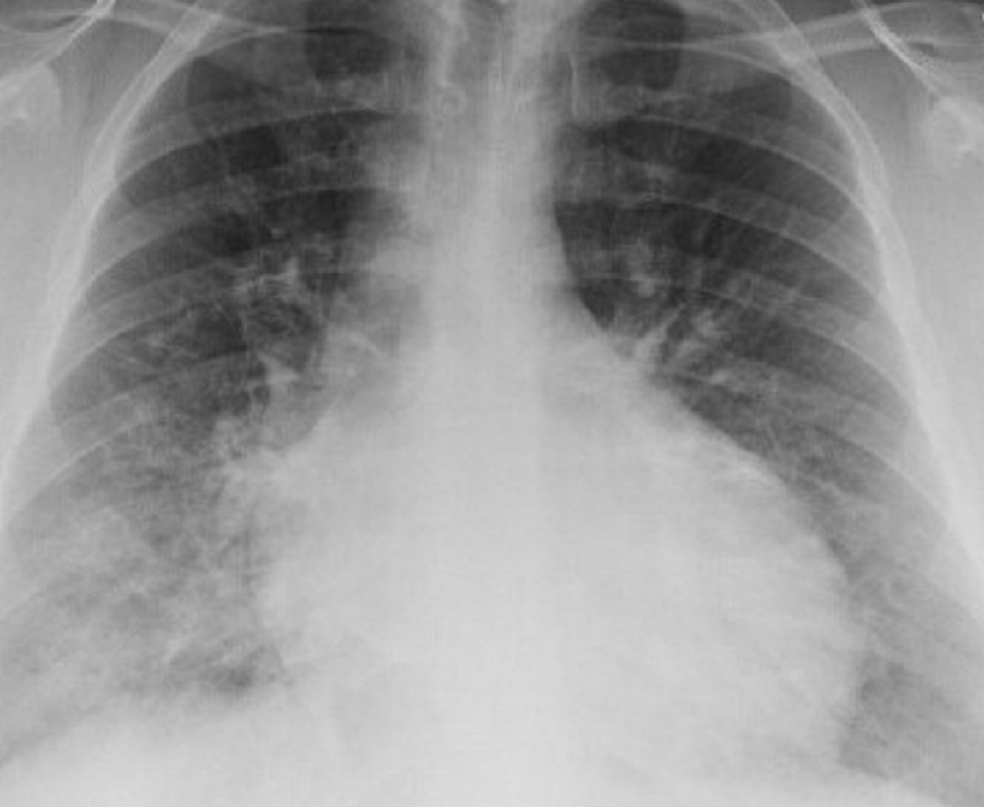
Chest X-ray showing bibasilar airspace opacities and cardiomegaly.
